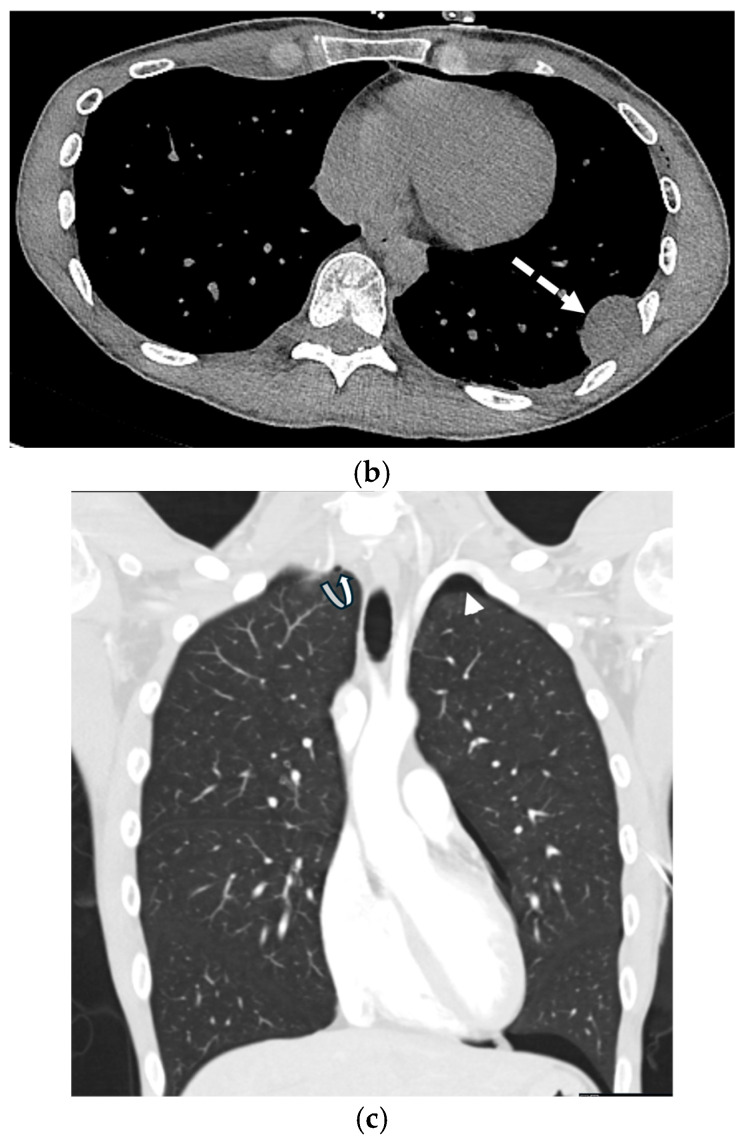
20-year-old male presents with SP. Frontal chest radiograph (a) shows moderate left pneumothorax (white arrowhead), along with pleural-based nodular opacity with an incomplete border sign in the inferior left hemithorax (dashed white arrow). Axial CT image in soft tissue window (b) demonstrates a well-circumscribed low attenuation lesion in the posterolateral left chest wall (dashed white arrow). Coronal CT image in lung window (c) reveals subpleural blebs in the right lung apex (curved white arrow) and small left pneumothorax (white arrowhead), status post-left chest tube placement. A subsequent surgical biopsy confirmed the diagnosis of neurofibroma.
